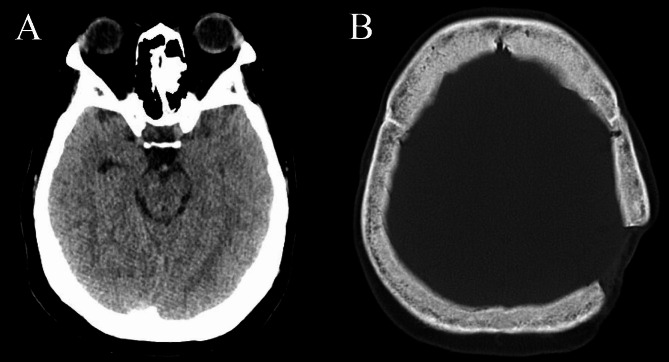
Head CT image showing a thickened skull base. A, Head CT image showed a thickened skull base; B, Head CT image showed a thickened skull base and the skull lesion caused by a previous traffic accident
